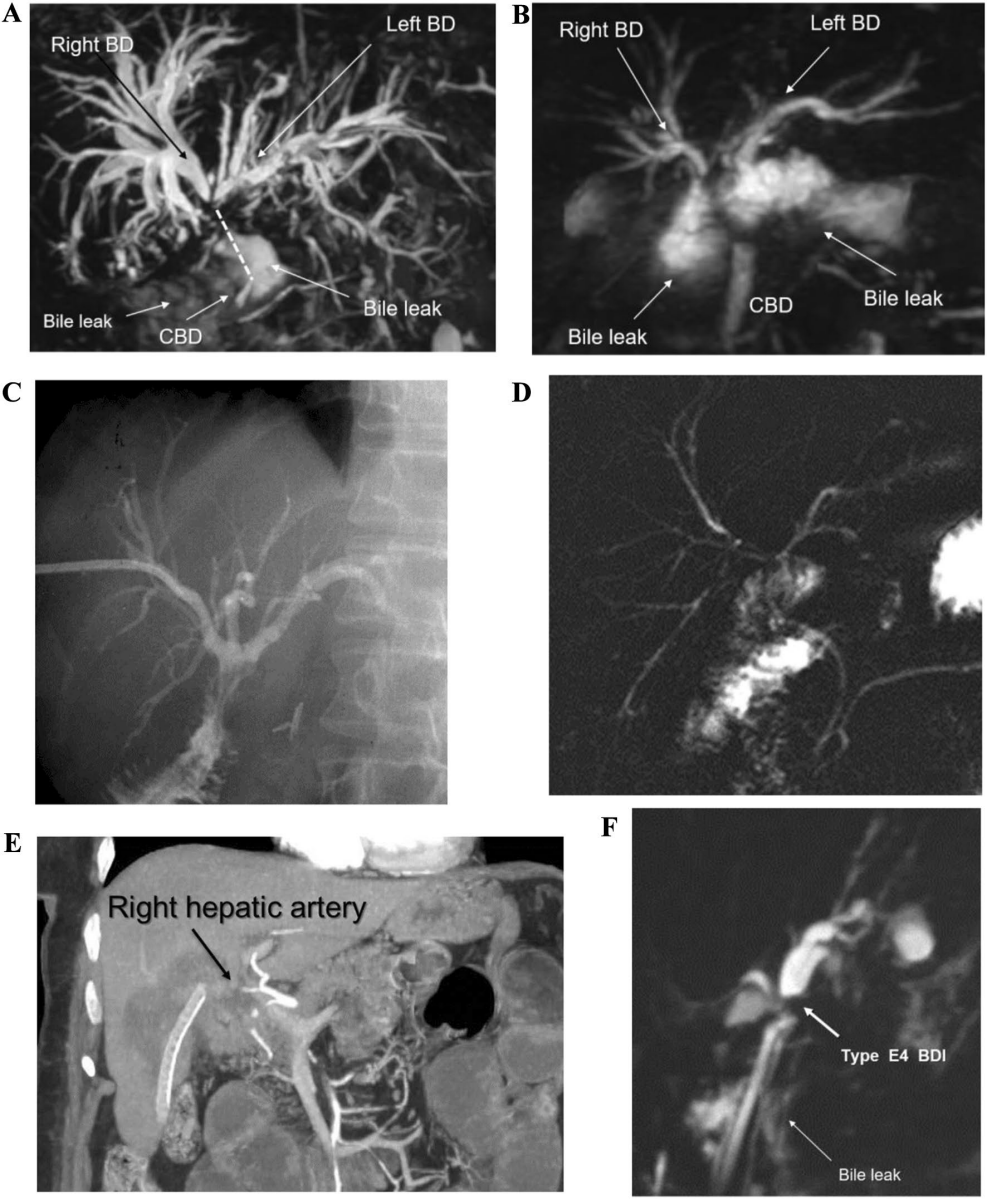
A MR cholangiography: Strasberg type E4 bile duct injury, with bile leak; the dotted line shows the long missing tract of the CBD. B MR cholangiography: Strasberg type E5 BDI, with bile leak. C Postoperative percutaneous cholangiography after HC-HJ. D MR cholangiography after HC-HJ. E, F MRI and MR cholangiography: right hepatic artery injury with Type E4 BDI with bile leak. *MR* magnetic resonance; *CBD* common bile duct; *BDI* bile duct injury; *HC-HJ* Hepp-Couinaud hepatico-jejunostomy; *MRI* magnetic resonance imaging
